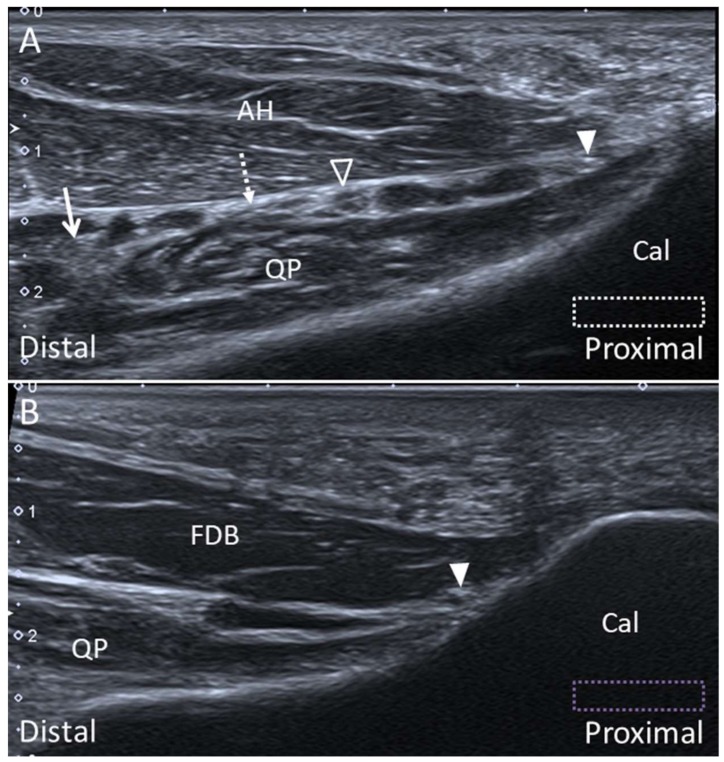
Ultrasound imaging of the Baxter nerve (arrowheads) between the abductor hallucis (AH) and the quadratus plantae (QP) muscles (A) and between the flexor digitorum brevis (FDB) and QP muscles (B). The colored dashed rectangles are in accordance with the transducer positions in Figure 3A,B. Lateral plantar nerve: void arrowhead; medial plantar nerve: arrow; medial septum: dotted arrow. Cal, calcaneus.
